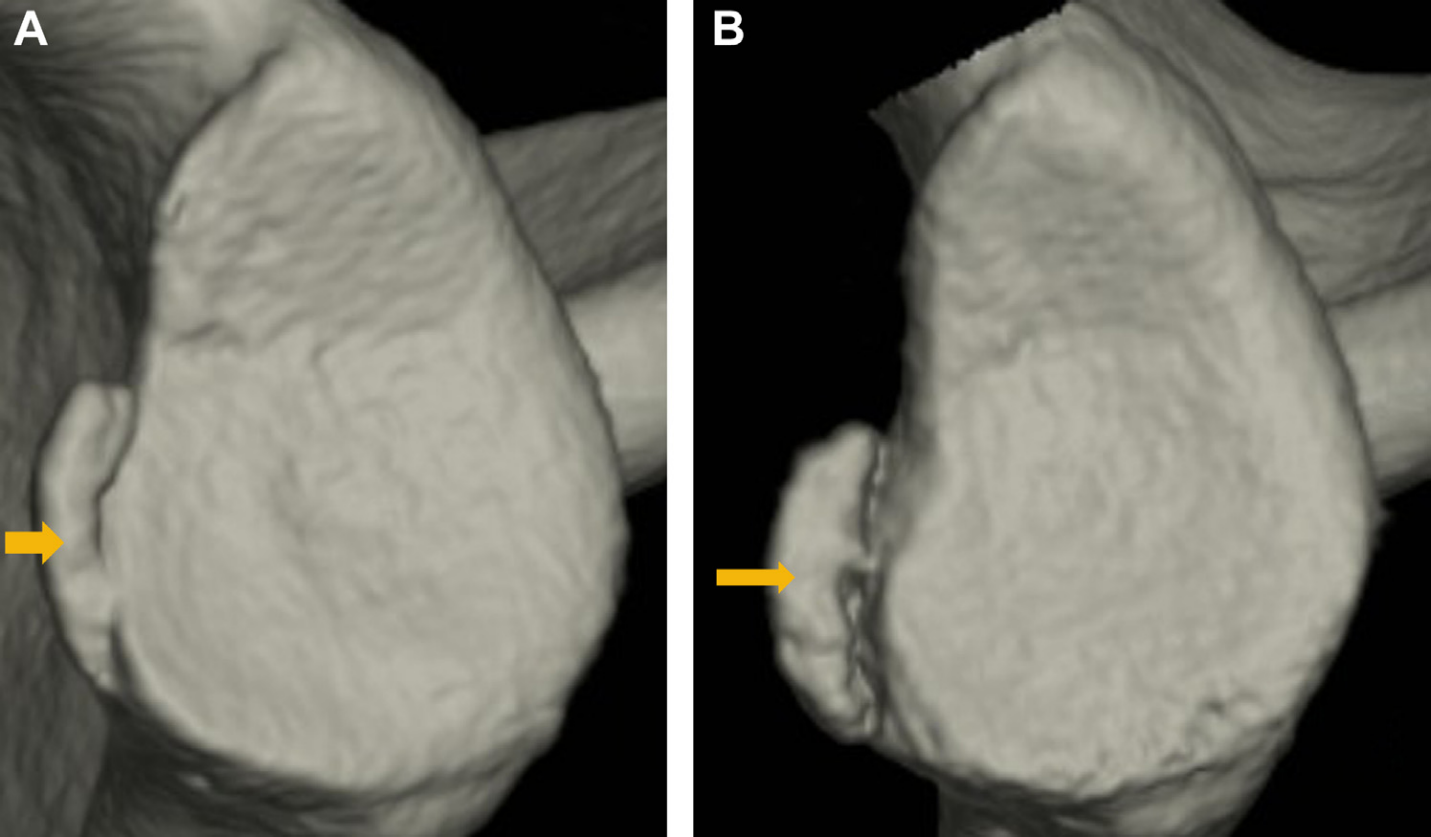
A male soccer player with an enlarged glenoid defect and enlarged bone fragment (left shoulder). (A): First computed tomography (CT) at primary instability (16 years old). (B): Third CT at 2 years after primary instability (18 years old, total 13 instability events). Both a glenoid defect and a bone fragment () increased in size from the first to third CT.
